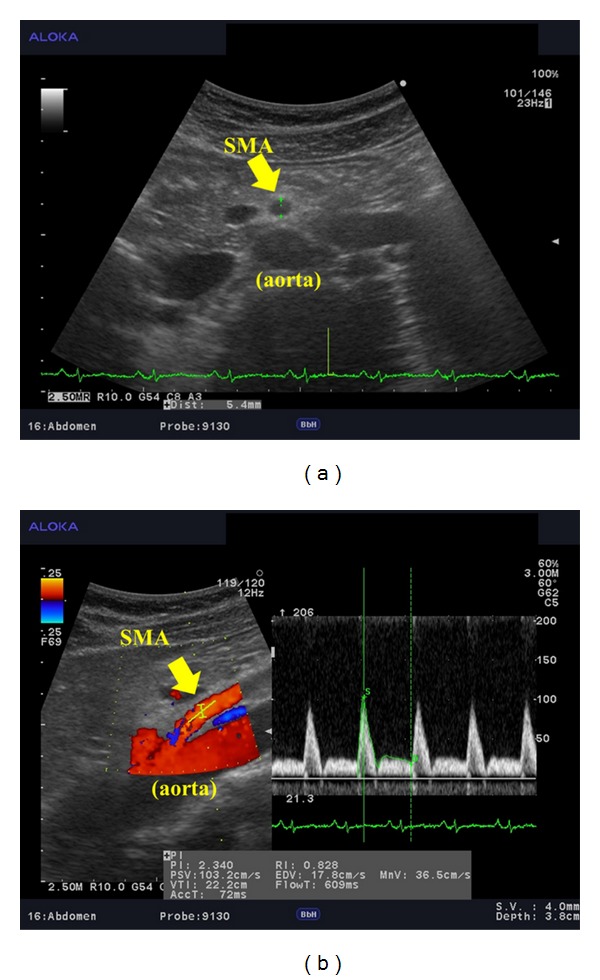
Haemodynamic data obtained by ultrasonography. (a) Measurement of the diameter of the superior mesenteric artery (SMA). (b) Measurement of the blood flow velocity in the SMA.
